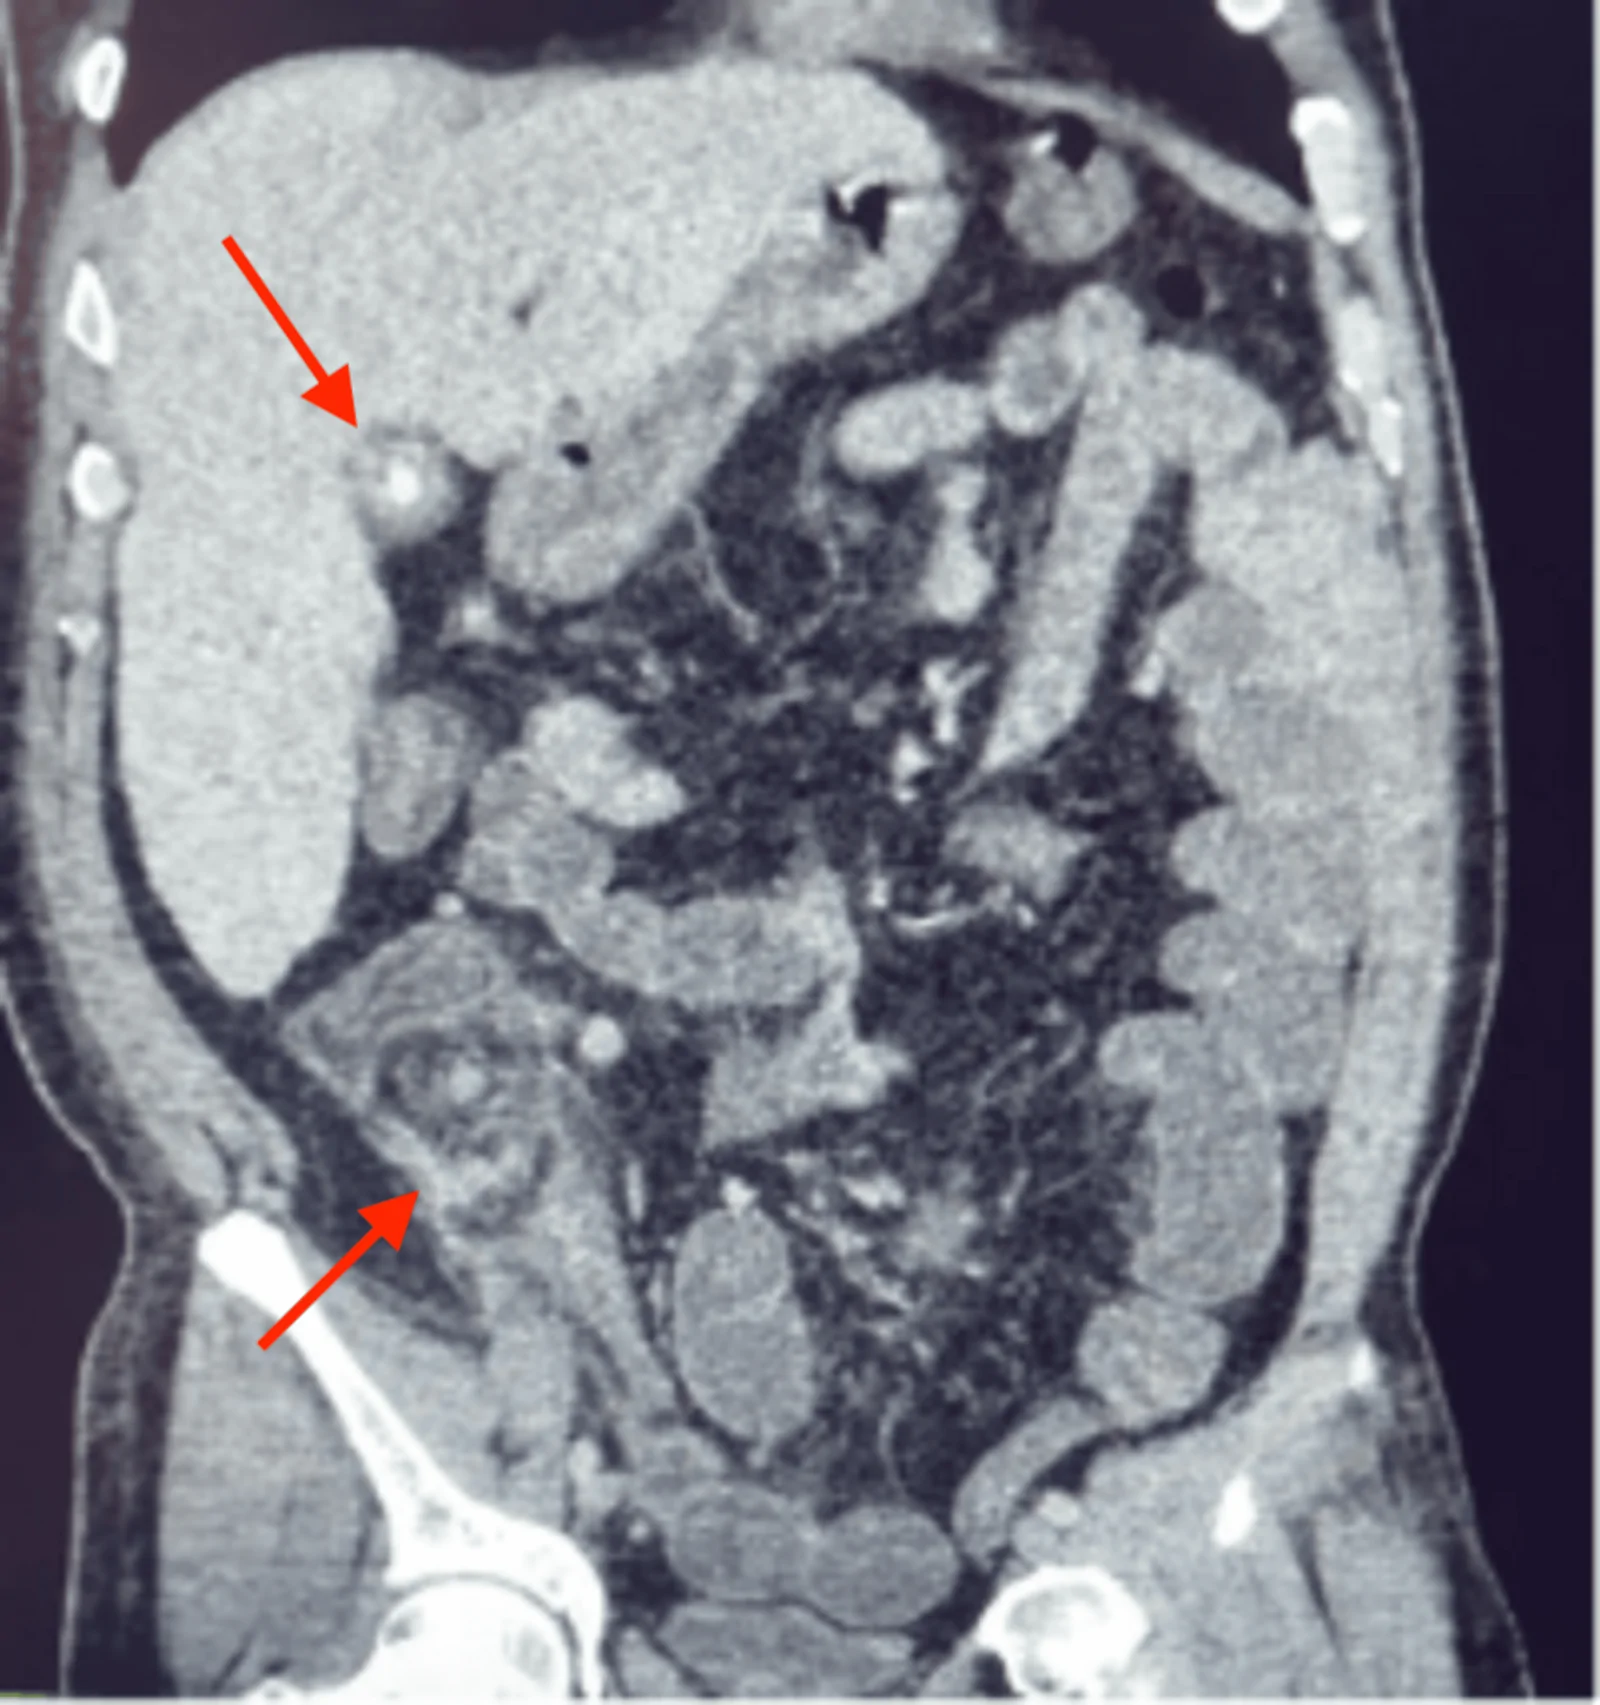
Synchronous view of the inflamed gallbladder and appendix on CT scan, modified coronal view. The upper arrow indicates the gallbladder with a stone and thickened wall. The lower arrow indicates the inflamed appendix.
